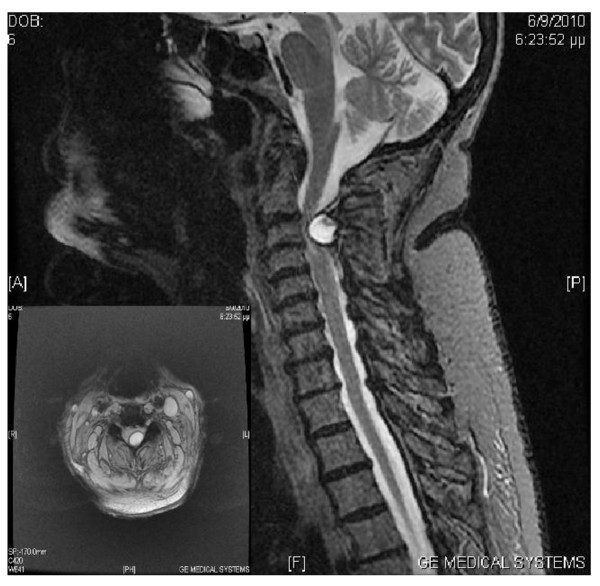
Preoperative magnetic resonance imaging. Mid-sagittal T2-weighted and axial T2-weighted images demonstrating an intracanalicular extradural space-occupying cystic lesion, which compresses and significantly displaces the spinal cord.
